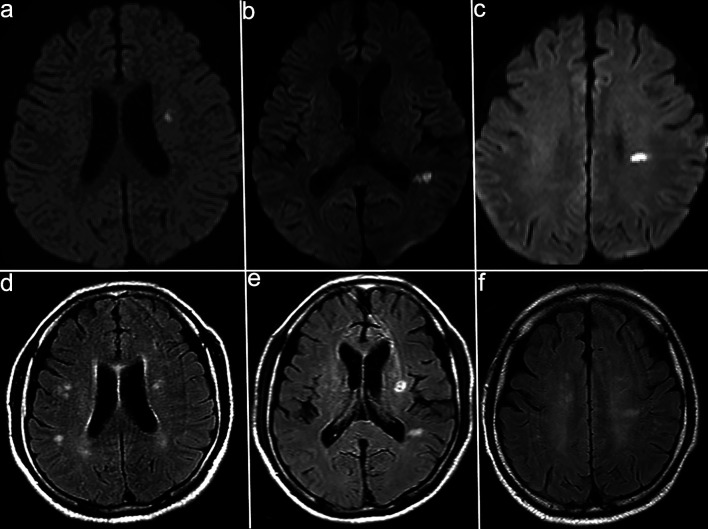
Serial diffusion-weighted imaging (DWI) showing the appearance of new lesions. (a–c) Follow-up magnetic resonance imaging (MRI) on day 7 (a), day 20 (b), and day 35 (c) shows small hyperintense lesions appearing sequentially in the left corona radiata and near the occipital left horn of the lateral ventricle. (d–f) Corresponding fluid-attenuated inversion recovery (FLAIR) images acquired at the same time points (a–d, b–e, and c–f pairs) demonstrate hyperintense white matter lesions.
